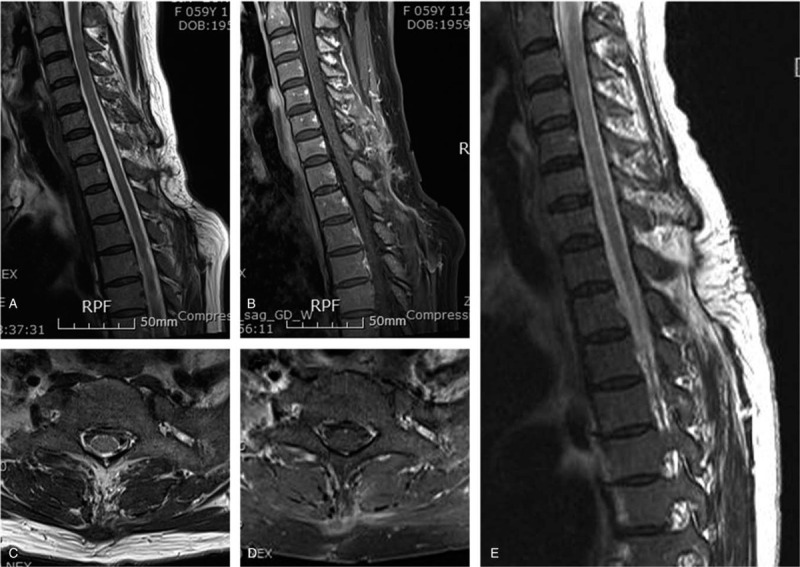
The preoperative symptoms improved immediately after the operation, and the magnetic resonance imagings conducted after 7 (A-D)- and 15 (E)-month postoperatively revealed no residual or recurrence lesion.
